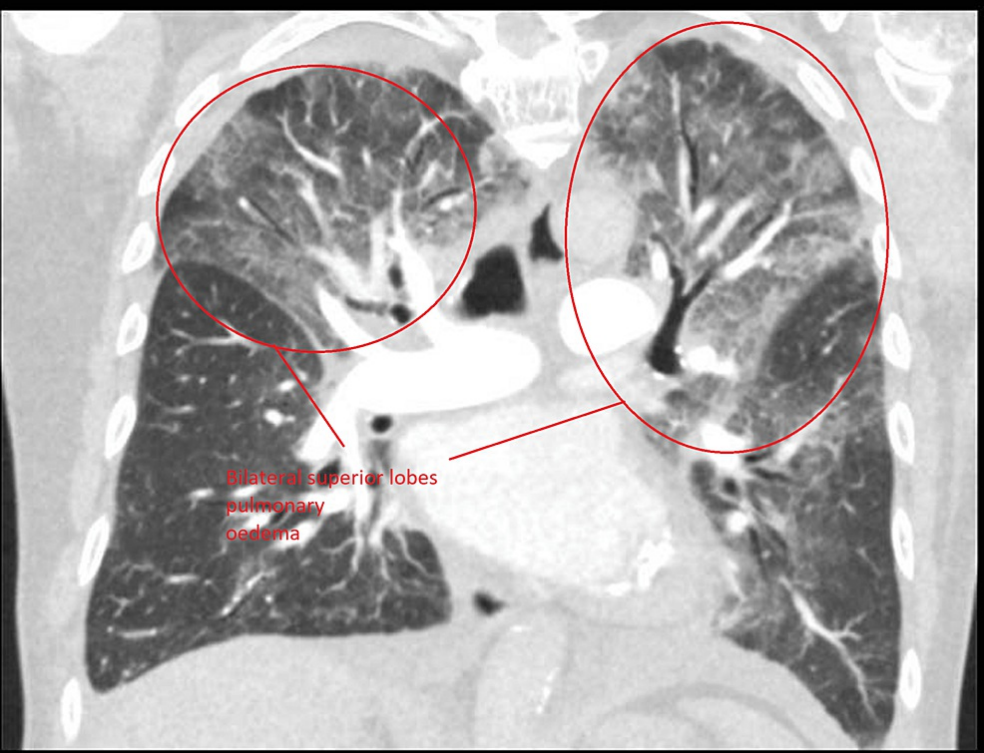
Computed tomography pulmonary angiogram. Computed tomography pulmonary angiogram did not show any pulmonary embolus but bilateral superior lobe pulmonary oedema.
